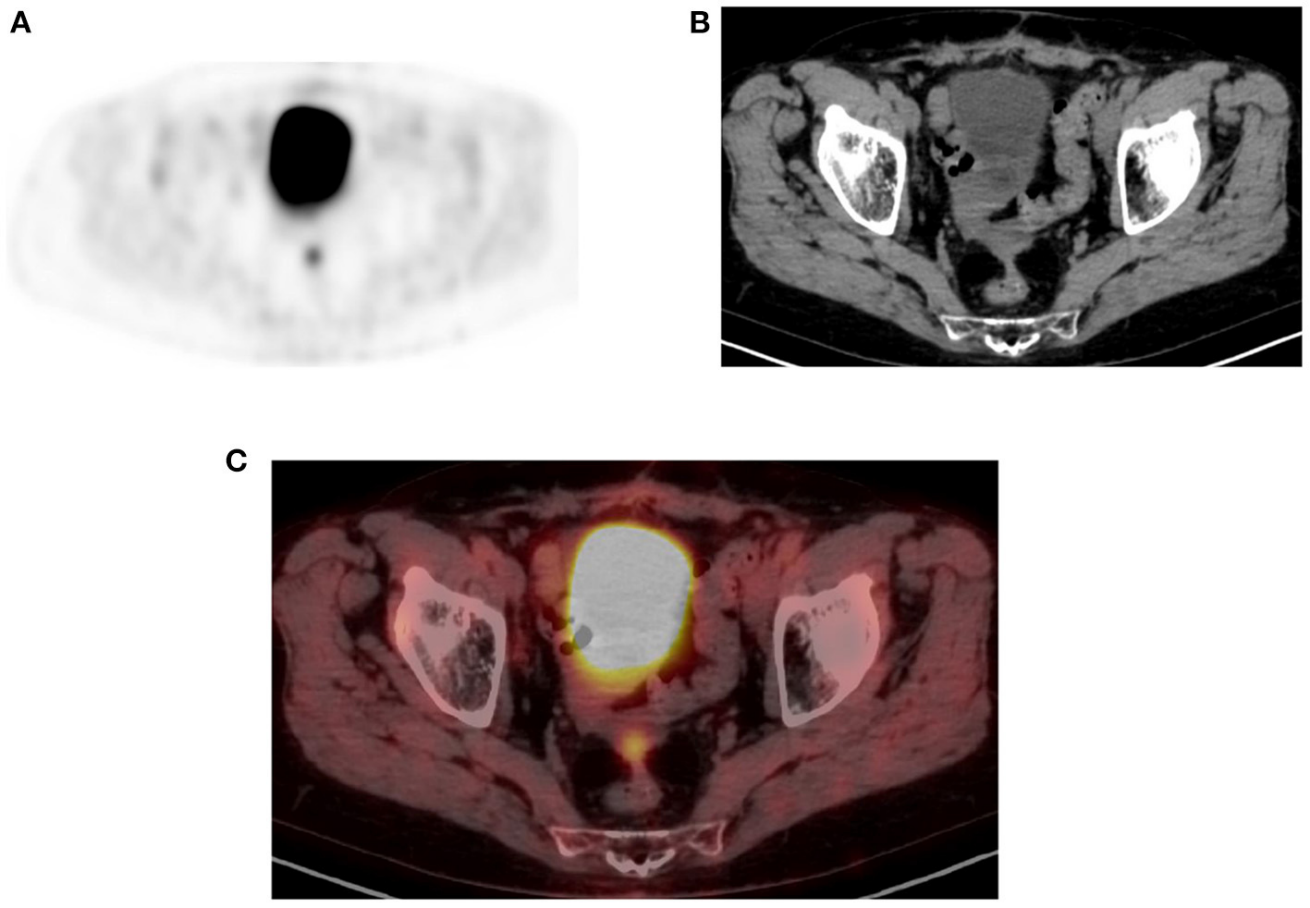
Physiologic pelvic focal uptake. FDG PET Axial (A) image showing focal increased uptake in posterior pelvic, in a 75-year-old female patient diagnosed with high grade serous carcinoma of the left ovary. She underwent oophorectomy and received chemotherapy. Her serum CA started to rise and was referred for restaging. The PET/CT showed active abdominal and pelvic adenopathy and in focal area of increased uptake that was localized in the vaginal fornix on CT (B) and combined PET/CT (C).
